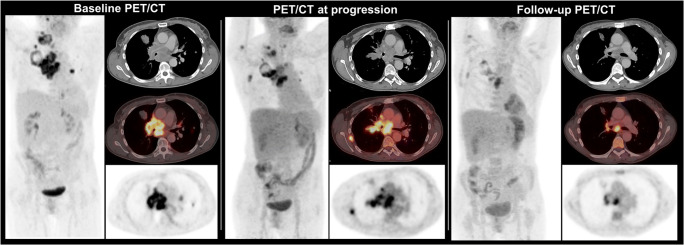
Treatment response assessment in a 49-year-old patient with NSCLC cT2b N3 M1 (TNM 8th edition) with extensive progressive disease (rib metastasis) 2 months after initiation of combined systemic treatment with carboplatin/pemetrexed/pembrolizumab. Consecutively, treatment was modified to carboplatin/paclitaxel/atezolizumab/bevacizumab; 8 weeks after initiation, a partial response was visible on FDG PET/CT with significantly decreasing metabolic tumor volume
